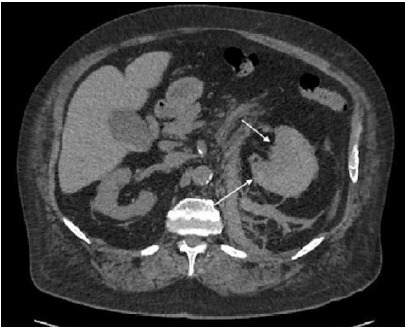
Computed tomography abdomen and pelvis without contrast showing subcapsular renal hematoma of the left kidney (white arrows).
